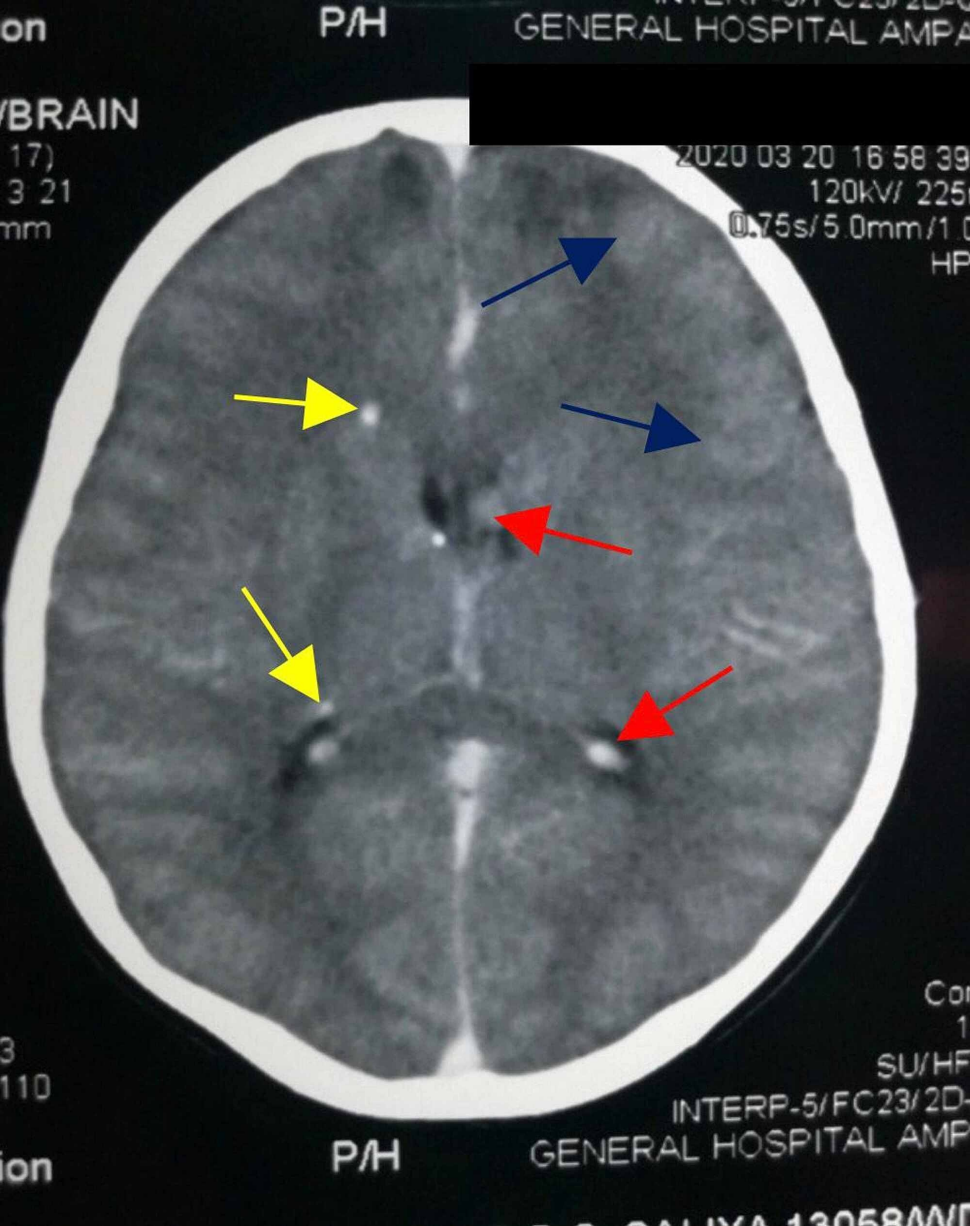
Contrast-enhanced CT showing cortical tubers in bilateral frontal and parietal regions (blue arrows), multiple partially calcified subependymal nodules (red arrows) and coarse calcifications (yellow arrows)
